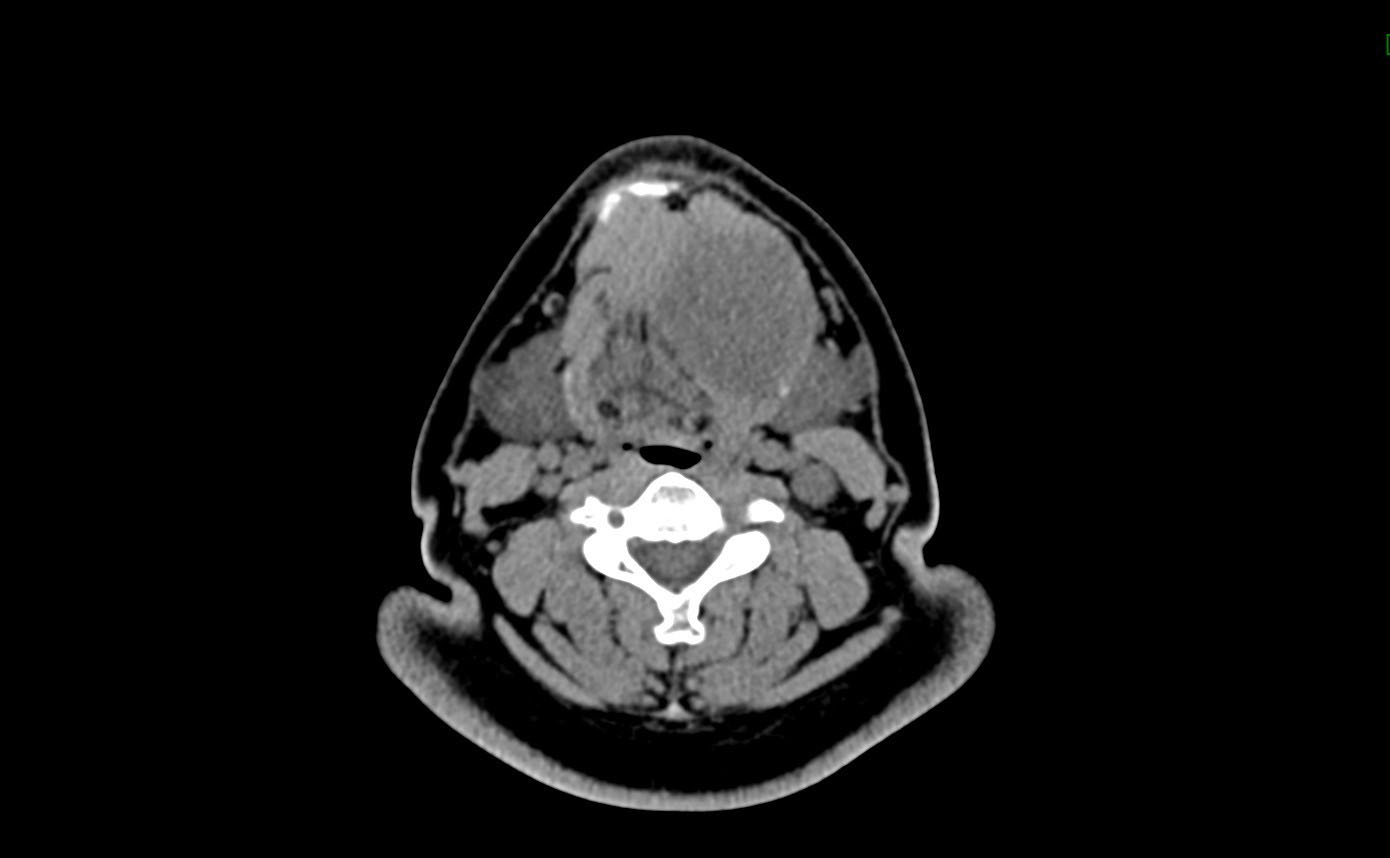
CT scan reveals a mass-like soft tissue density shadow in the left submental region, measuring approximately 53×50×49 mm.
